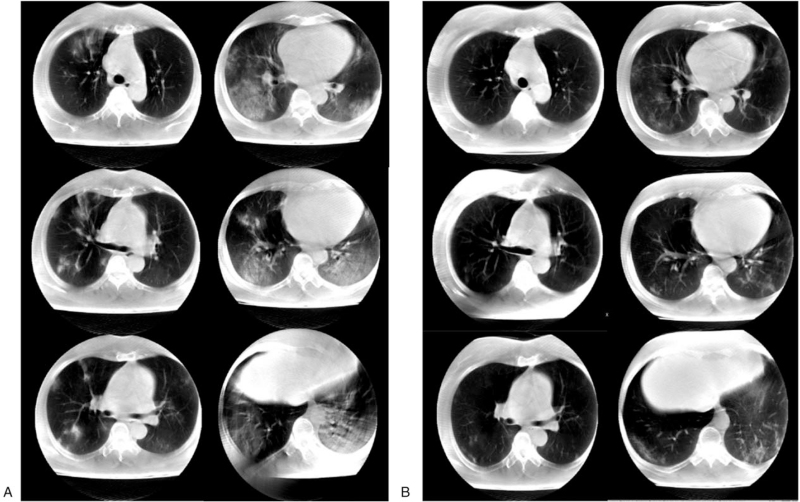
Mobile CT scan in patients with COVID-19 pneumonia. (A) The initial axial CT scans revealed multifocal ground-glass opacities and mixed consolidation distributed from peripheral to subpleural in both lungs. (B) Evidence of apparent resolution of the parenchymal lesions was identified in follow-up axial CT scans obtained on hospital day 9. COVID-19 = coronavirus 2019, CT = computed tomography.
